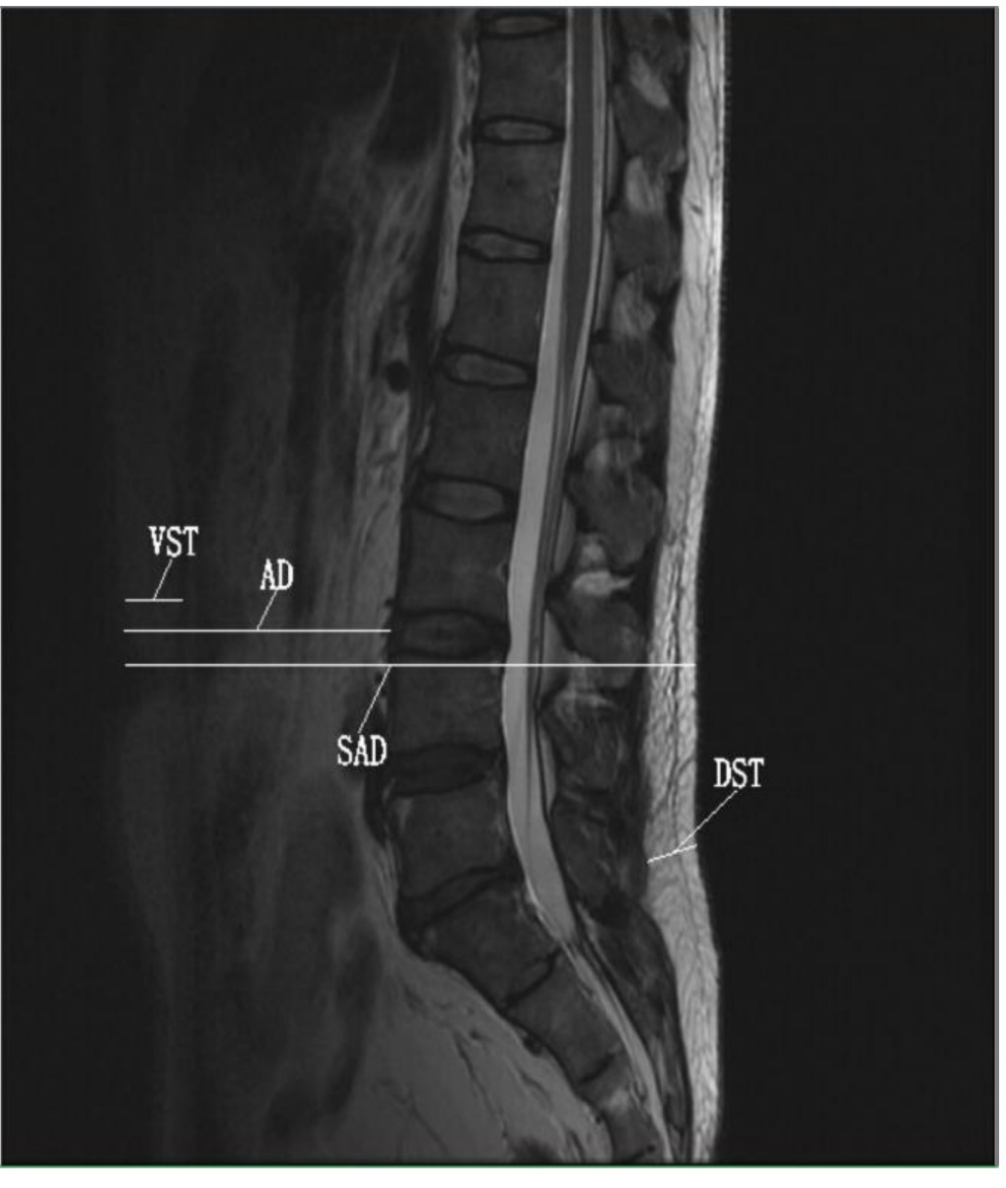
T2-weighted midsagittal plane images of the lumbar spine showing the adiposity diameters measured Abbreviations: VST, ventral subcutaneous thickness; AD, abdominal diameter; SAD, sagittal diameter; DST, dorsal subcutaneous thickness.
